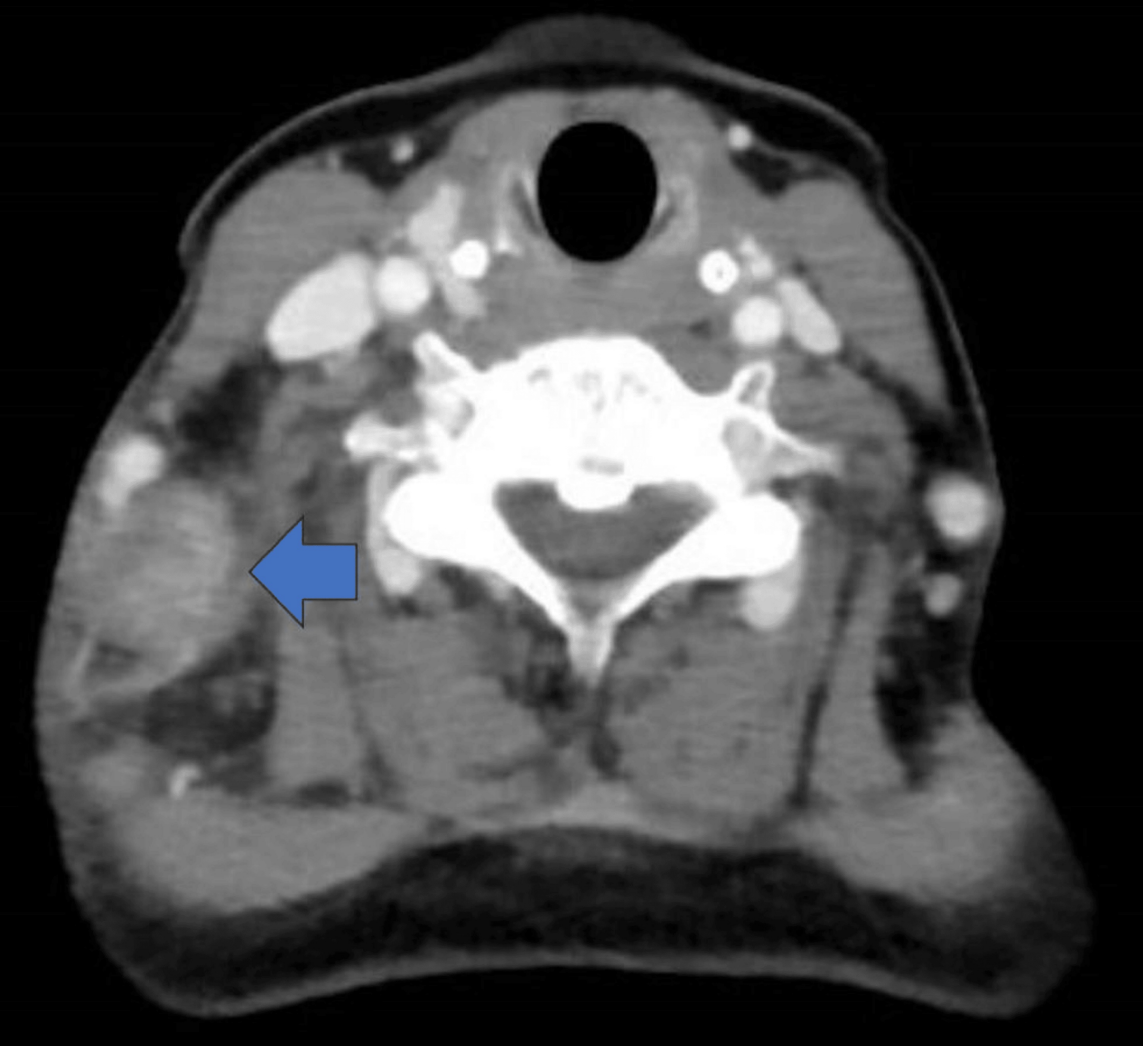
CT image of the neck. Contrast-enhanced cervical CT demonstrating enlargement of the right cervical lymph node (arrow). Axial view; slice thickness 5 mm.
